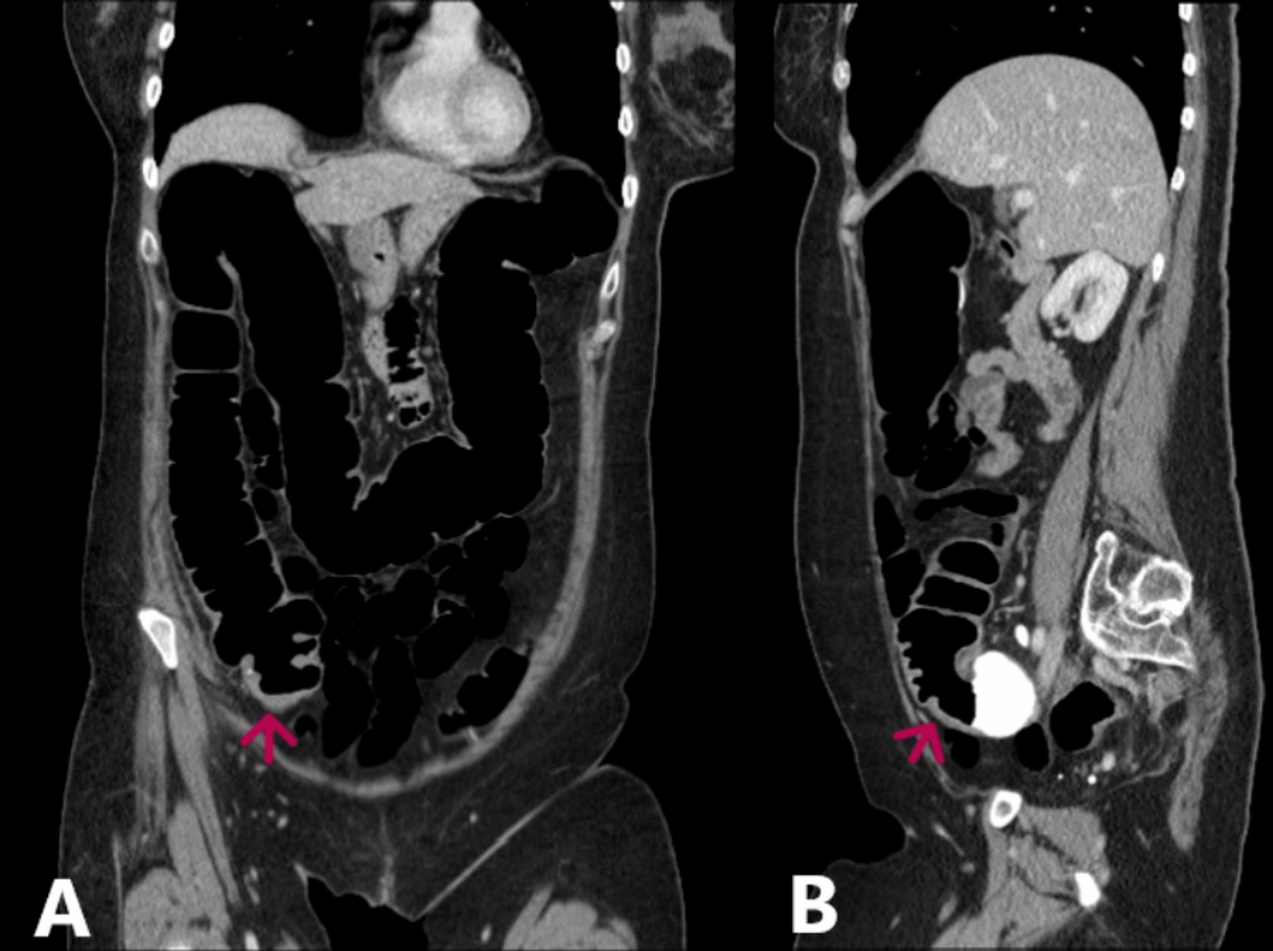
CT colongram shows moderately tortuous colon with suspicious caecal wall thickening (red arrows) CT: Computed tomography
